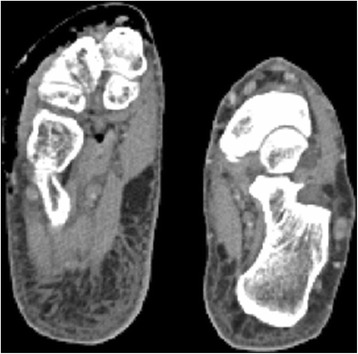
A CT scan showed extension of the emphysema to the toes
